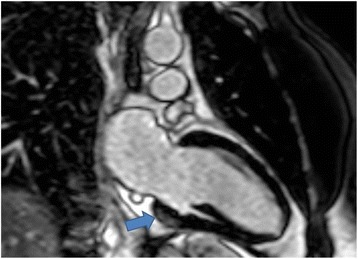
Cardiac magnetic resonance imaging of patient showing myocarditis. The arrow shows sub epicardial delayed gadolinium enhancement in the basal to inferolateral segment of the heart
